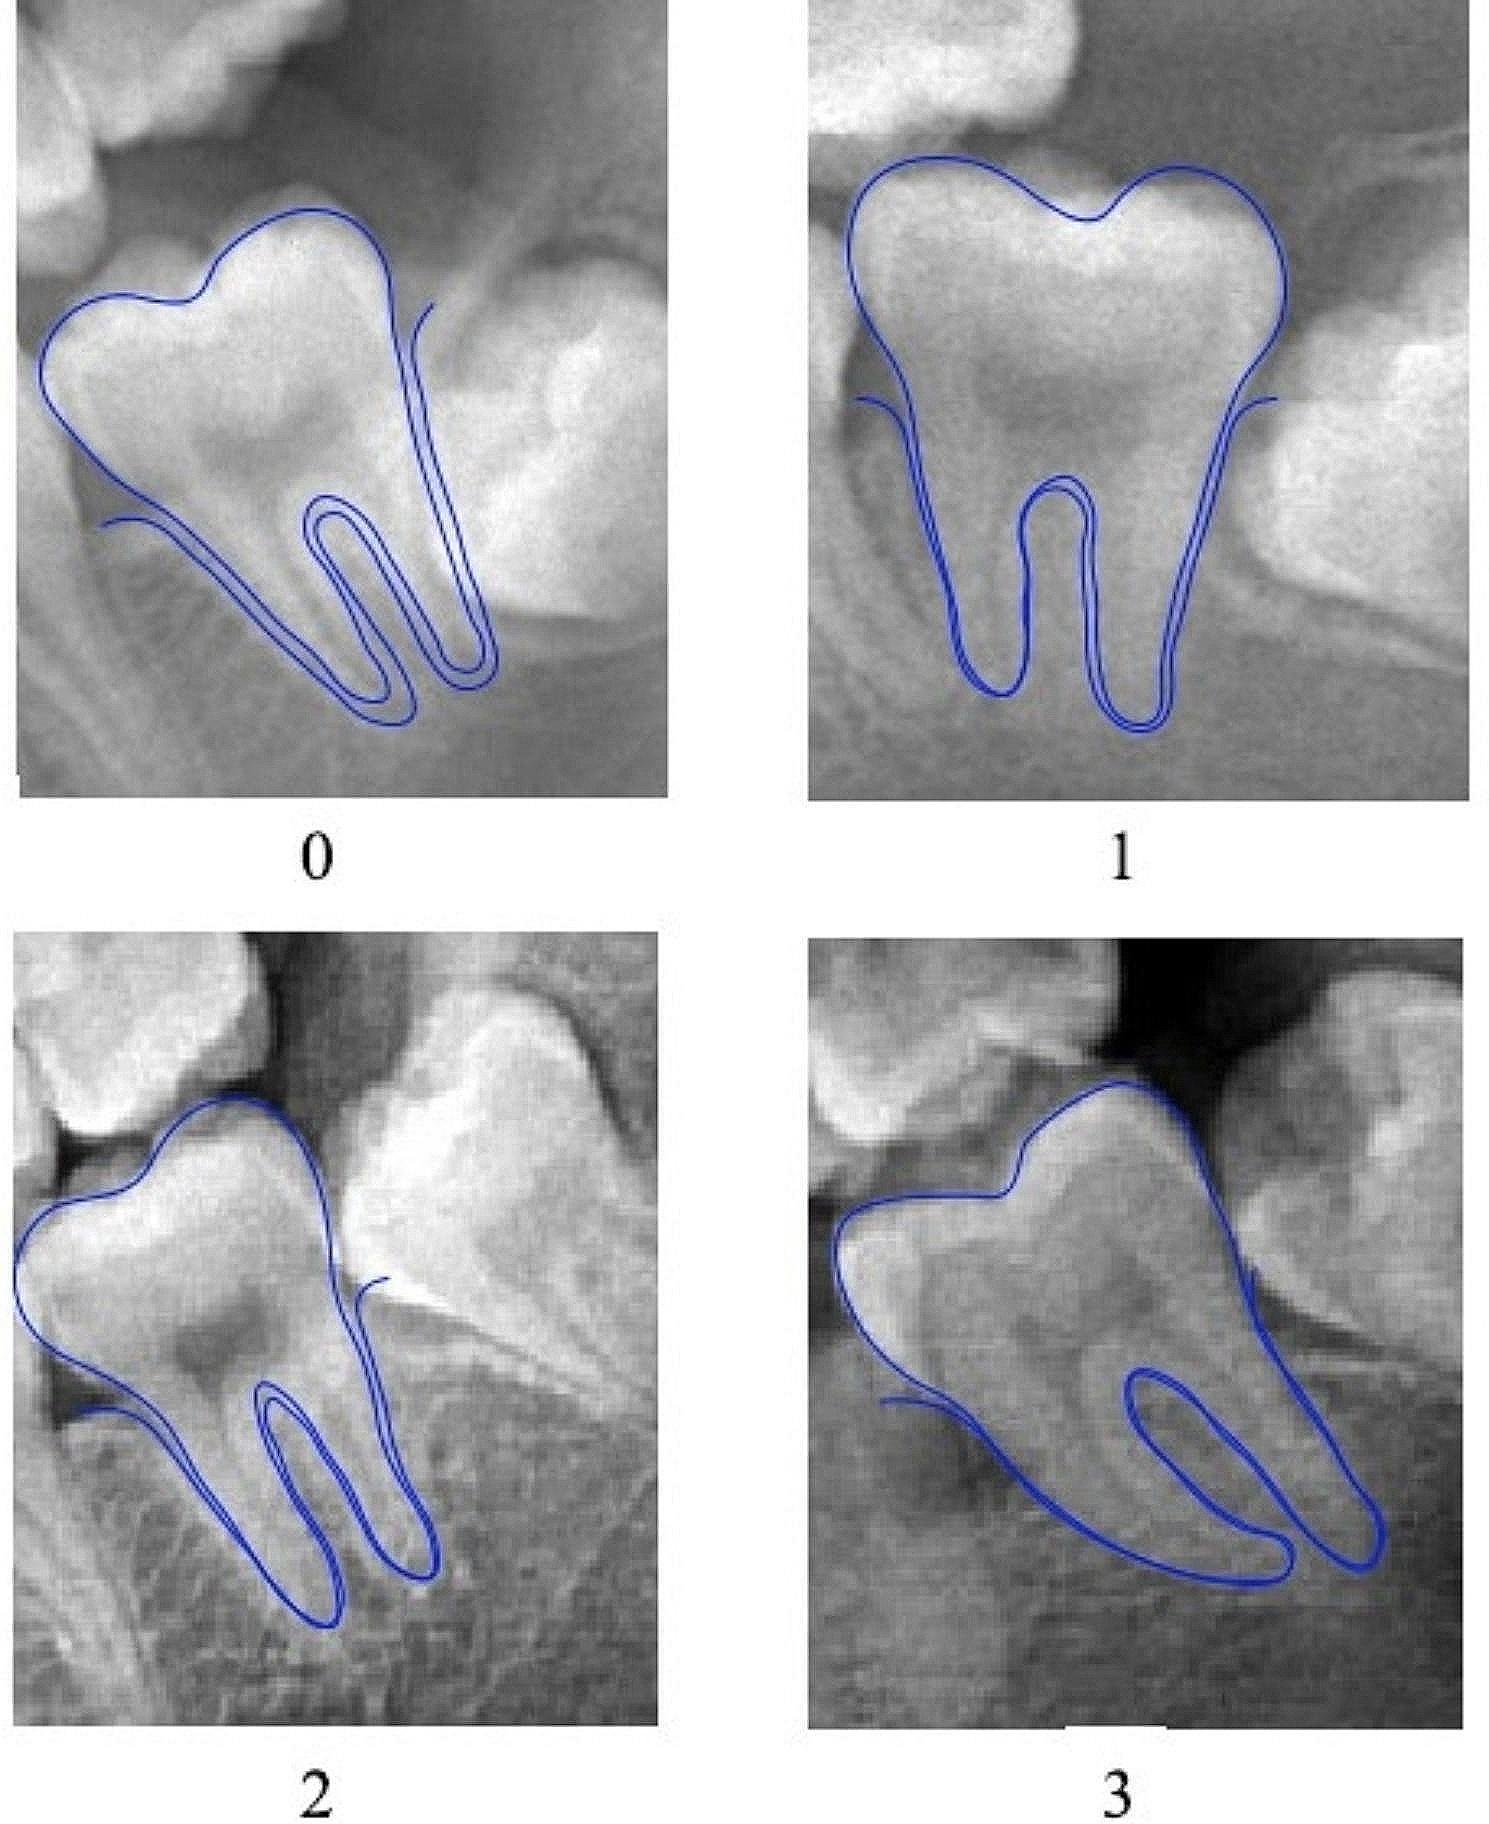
Pictures of the stages of radiographic visibility of the periodontal ligament in second molars
